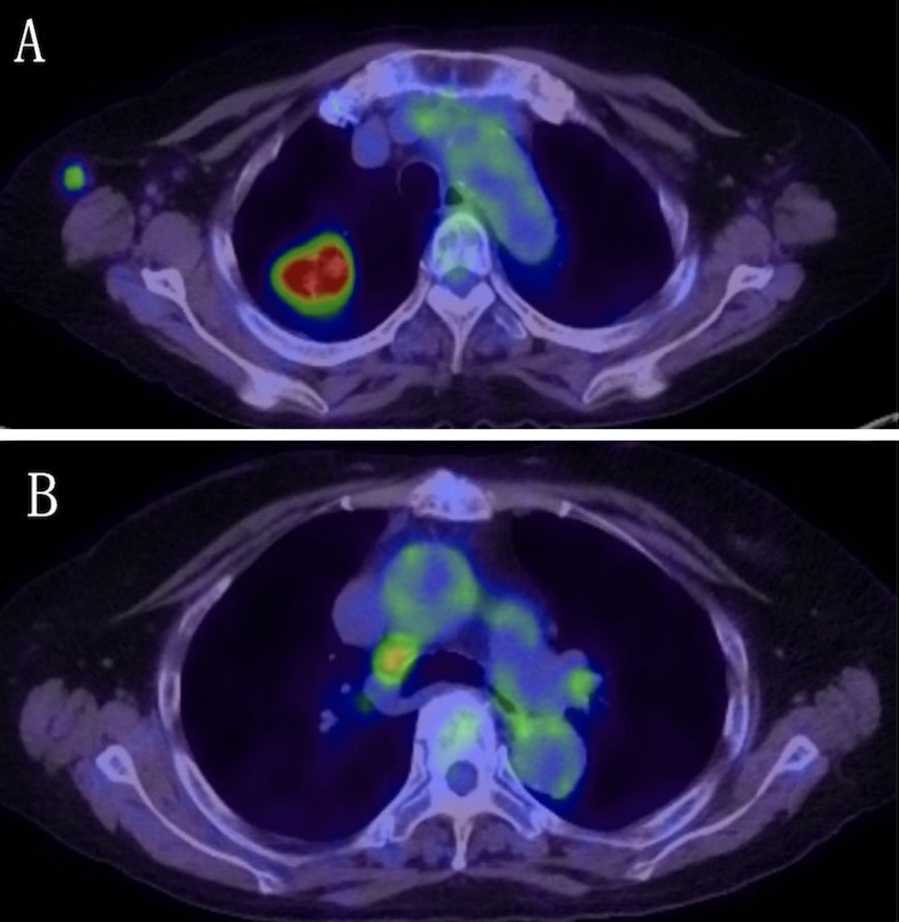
A 18F-fluorodeoxyglucose (FDG) positron emission tomography scan shows FDG accumulation with a maximum standardized uptake value (SUVmax) of 11.78 in the mass and B FDG accumulation with a SUVmax of 3.48 in the right lower paratracheal lymph node (station #4R)
